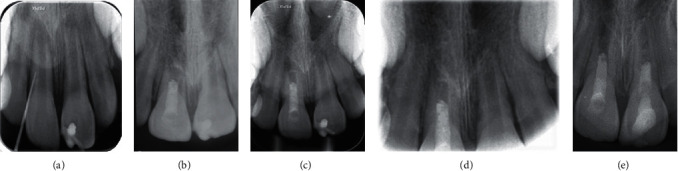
(a) Periapical radiograph of the maxillary right central incisor with traced mucosal sinus tract using a no. 30/0.02 gutta-percha point. (b) Immediate postoperative radiograph showing completion of RET. (c) Two-month follow-up. (d) Four-month follow-up. (e) Fourteen-month follow-up.
